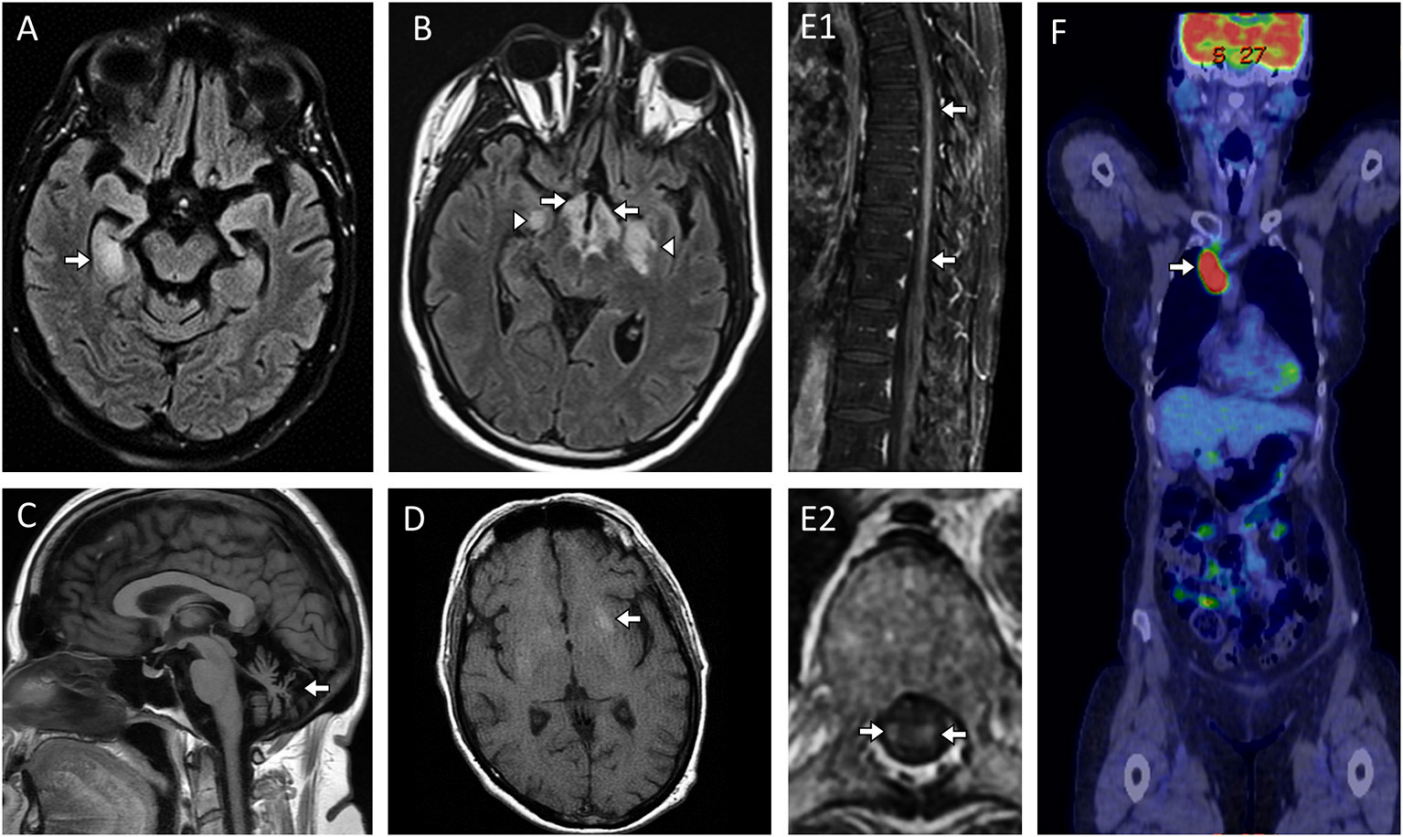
MRI abnormalities in patients with paraneoplastic and other non-demyelinating antibody-mediated CNS disorders. (A) Paraneoplastic limbic encephalitis with antineuronal nuclear antibody type 1 (ANNA-1/anti-Hu) with MRI head axial FLAIR image revealing unilateral T2-hyperintensity in the right mesial temporal lobe [(A), arrow]; (B) Paraneoplastic narcolepsy-cataplexy and limbic encephalitis with Ma-2/Ta antibodies and MRI axial FLAIR image revealing bilateral T2-hyperintensity in the hypothalamus [(B), arrows] and bilateral mesial temporal lobes [(B), arrowheads]; (C) Progressive cerebellar ataxia with neurochondrin autoantibodies and MRI head sagittal T1-weighted images without gadolinium revealing severe cerebellar atrophy [(C), arrow]; (D) Facio-brachial dystonic seizures with leucine-rich-glioma-inactivated-1 (LGI1) antibodies with MRI head axial T1-weighted images without contrast administration revealing T1-hyperintensity in the left basal ganglia [(D), arrow]; (E) Paraneoplastic myelopathy accompanying small cell lung cancer without an identified neural autoantibody with MRI spine T1-weighted sagittal and axial images with gadolinium revealing enhancement within the spinal cord parenchyma [(E1, E2): arrows] which on axial images was restricted to the bilateral lateral columns [(E2), arrows) which is a hallmark imaging finding with paraneoplastic myelopathy; (F) Paraneoplastic encephalitis with α-amino-3-hydroxy-5-methyl-4-isoxazolepropionic acid (AMPA) receptor antibodies and 18F-Flludeoxyglucose-positron-emission-tomography (FDG-PET) body revealing markedly increased glucose uptake in the right paratracheal region [(F), arrow] with subsequent biopsy revealing metastatic small cell lung cancer.
